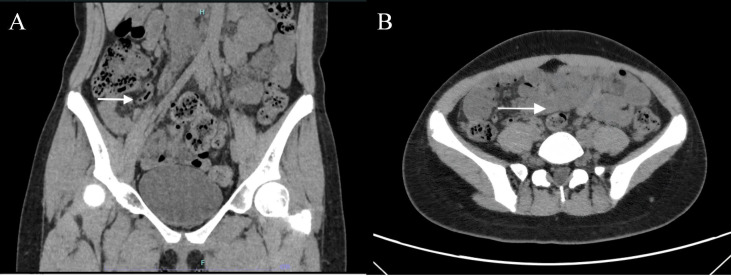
(A) Abdominal CT imaging demonstrated appendiceal wall thickening, luminal gas, and peri-appendiceal fat stranding, accompanied by multiple enlarged lymph nodes in the ileocecal region (white arrow); (B) Abdominal CT revealed nodular mesenteric masses (white arrow).
